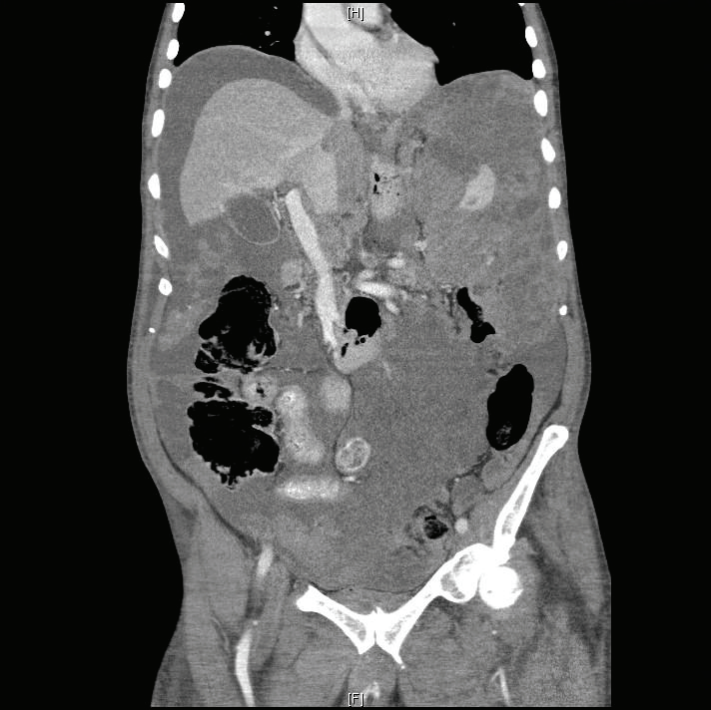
Coronal CT view demonstrating marked omental caking with interspersed high density fluid filling the left hypocondrium and left peritoneal region.
